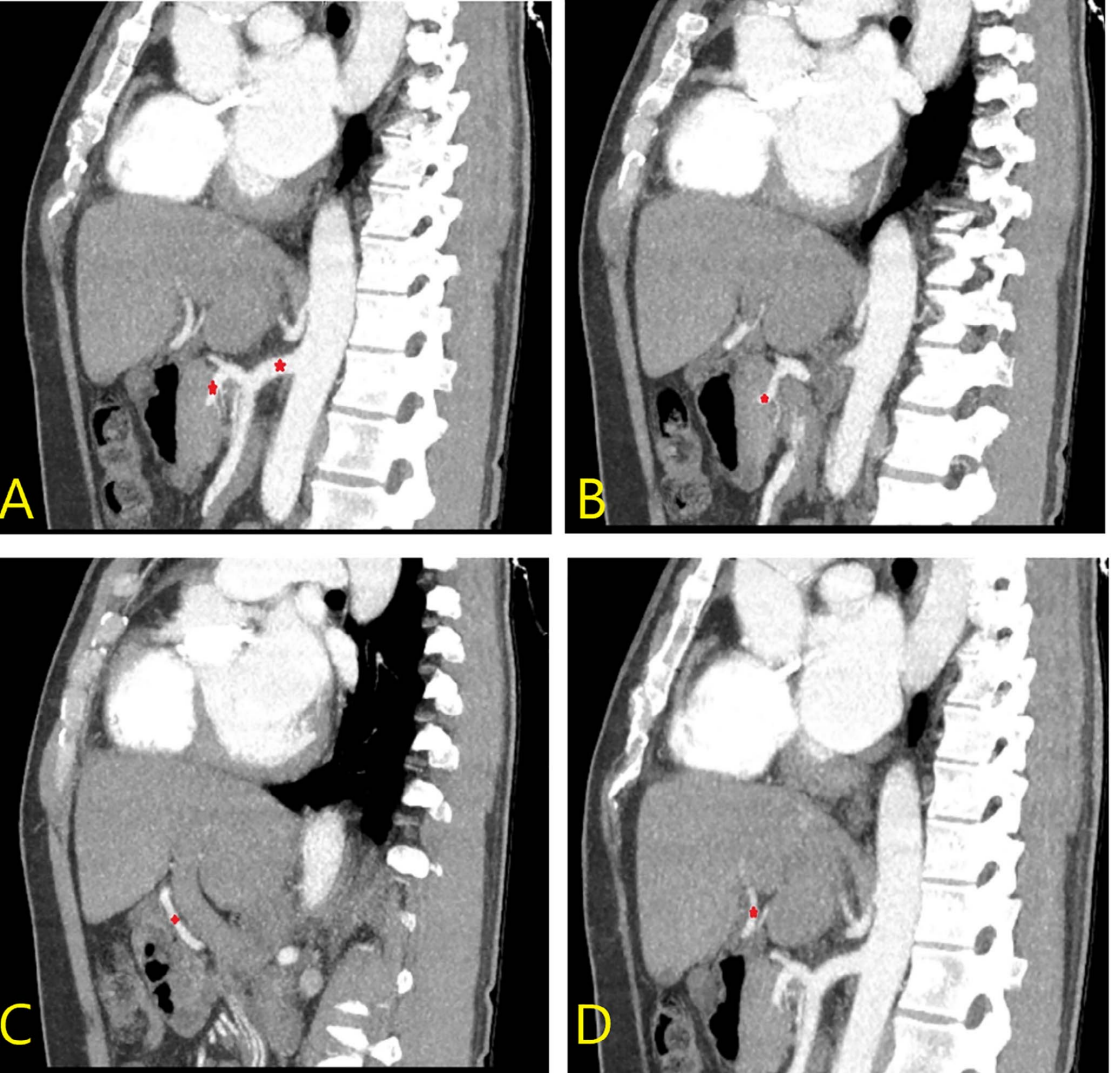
Thin-section (1 mm), sagittal multiplanar reformatted contrast-enhanced computed tomography images show in arterial phase (A) the common hepatic artery branch originating from the superior mesenteric artery, and in consecutive sections (B, C, D), the course of the common hepatic artery toward the liver, consistent with the variant hepatic arterial anatomy. In all images, the common hepatic artery is marked with a red star.
